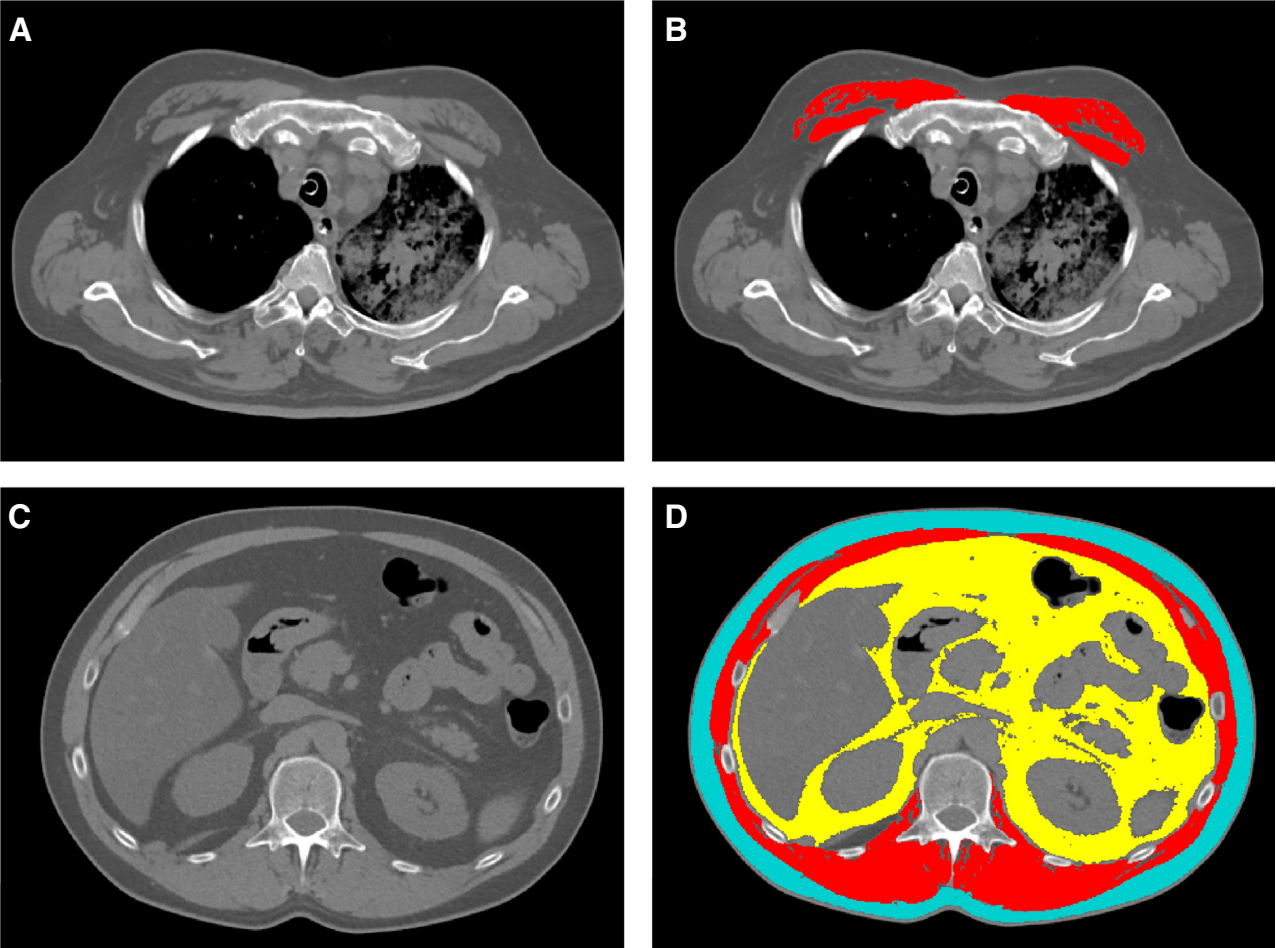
A-D, Representative examples of selected CT scan slices at the fourth thoracic vertebra level (A) with demarcated pectoralis major and minor muscle (B) and at first lumbar vertebra (L1) level (C) with demarcated L1 muscle, visceral, and subcutaneous adipose tissue (D).
